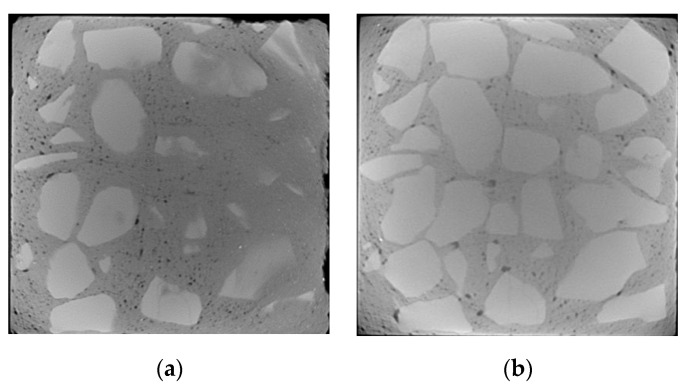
CT images of D5 before sulfate attack (no cracks): (a) surface layer; (b) inner layer.
